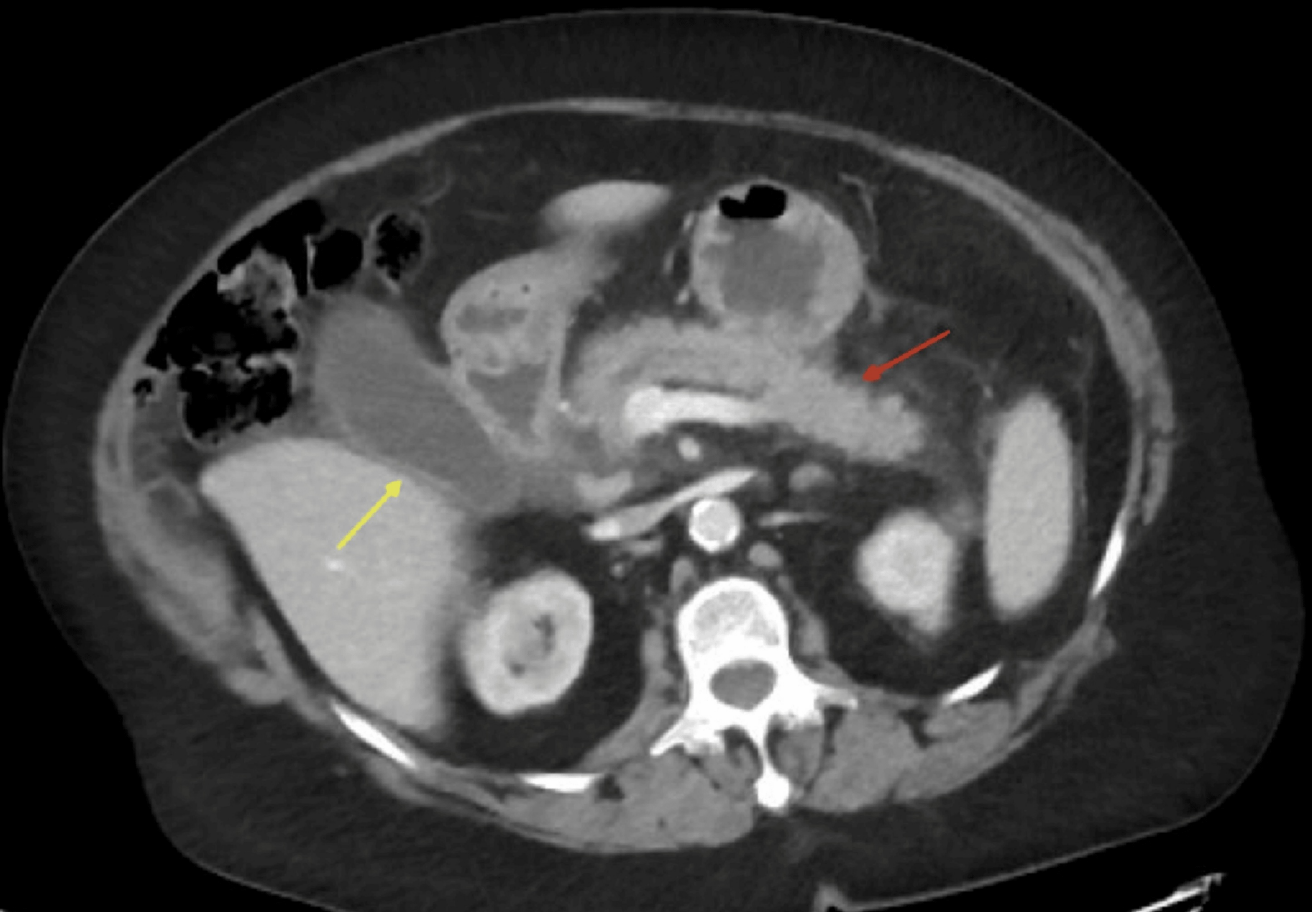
Axial abdominal CT scan showing the distended gallbladder with thickened wall (yellow arrow) and inflamed pancreas with peripancreatic stranding (red arrow) consistent with acute pancreatitis CT: computed tomography
